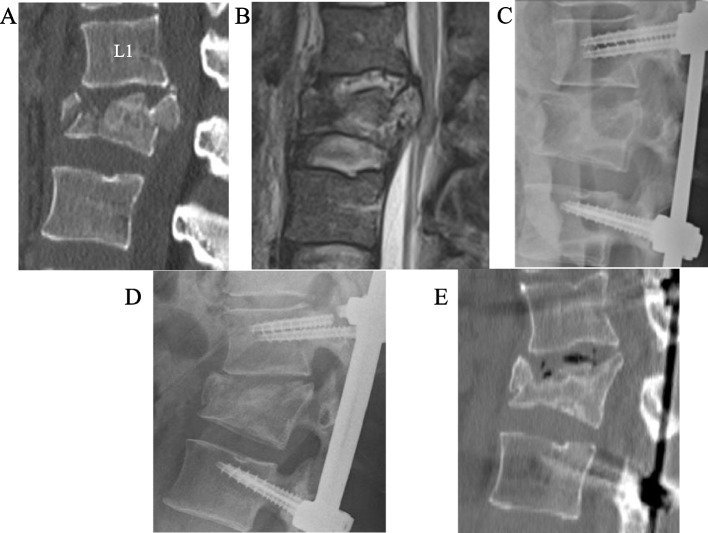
A 39-year-old woman with L2 burst fracture (AO A3). CT (A) and MRI (B) showed severe damage of the cranial endplate and infraction of the disc into the vertebral body (TIDL grade 3). The fractured vertebra was reduced after surgery (C). At follow up, fractured vertebra showed bony union, however disruption of the vertebral endplate and degeneration of intervertebral disc resulted in correction loss and breakage of the pedicle screw (D, E). Panel E shows residual endplate deformity
